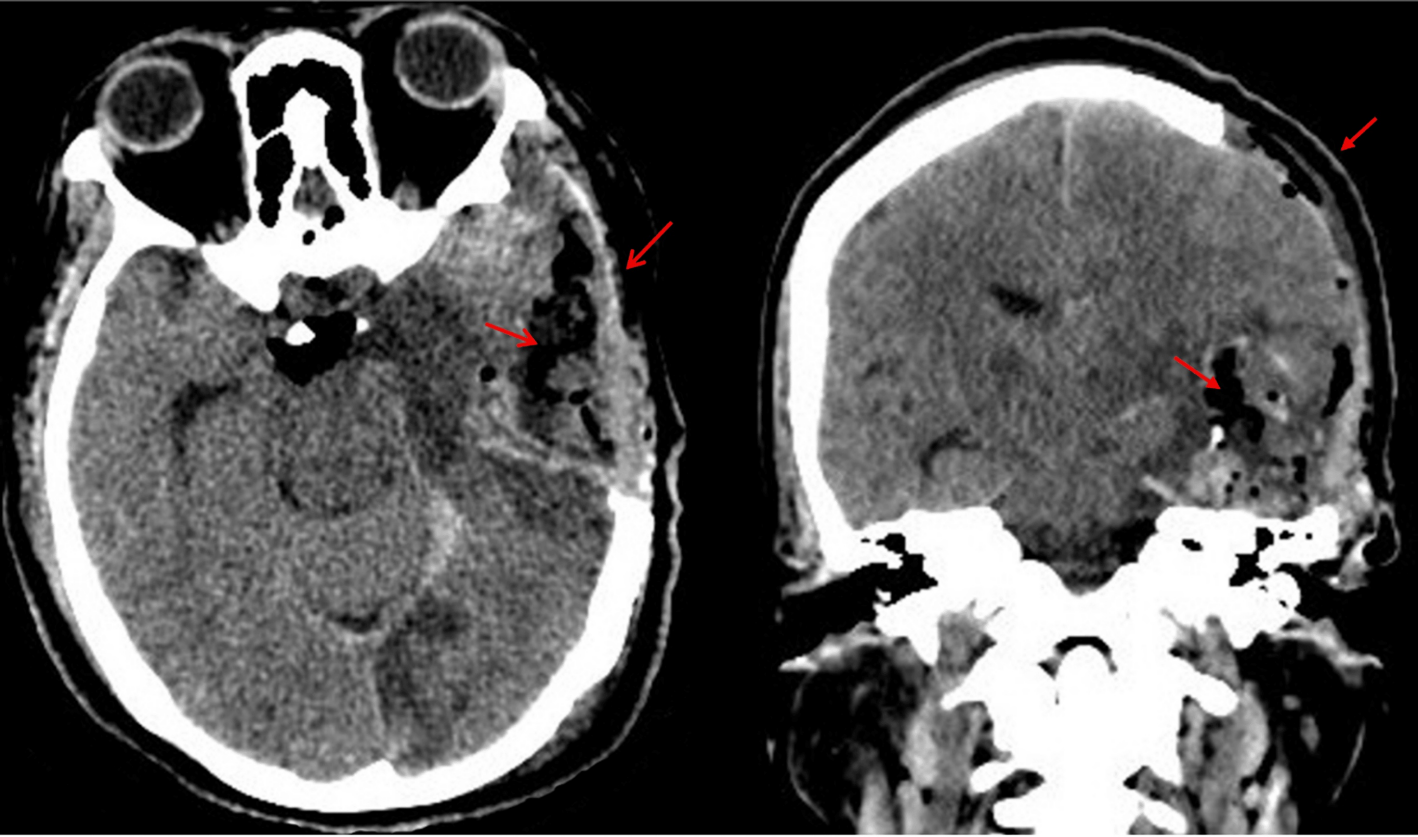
Postoperative non-contrast skull computed tomography scan showing no residual injury Follow-up axial and coronal non-contrast brain computed tomography showing postoperative changes in the left fronto-temporo-parietal region, with a surgical cavity, residual pneumocephalus, resolution of the previously noted midline shift, and no evidence of residual hematoma or acute intracranial hemorrhage (red arrows).
